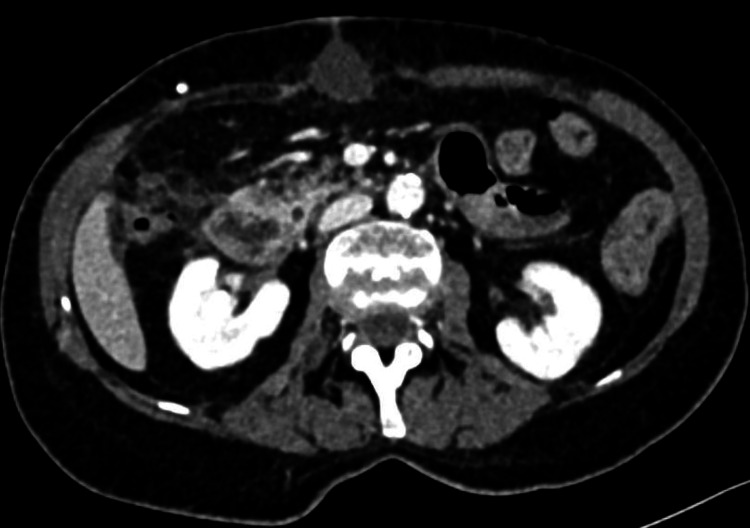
Postoperative CT of the abdomen and pelvis. Normal duodenal wall without periduodenal fluid collection.
